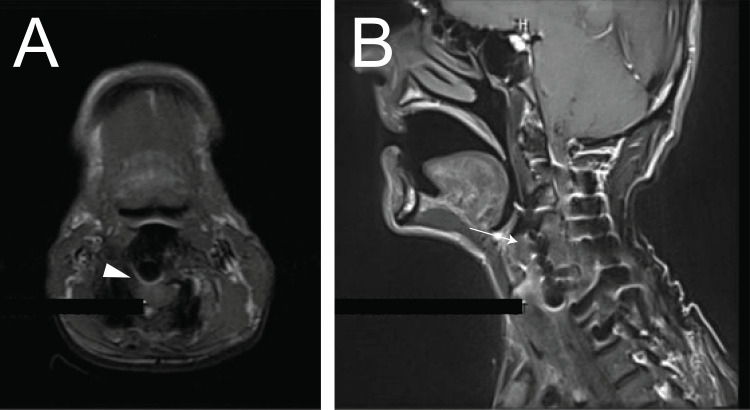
Neck MRI. The absence of expected soft tissue with the posterior wall is suggestive of hardware erosion (arrowhead- 1A). There is an abnormal thickening of the laryngeal soft tissues, with medialization of the right vocal cord and loss of expected parapharyngeal fat (arrow- 1B).
